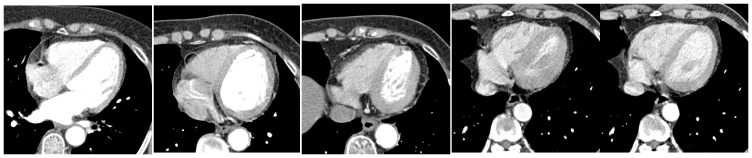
Some examples of cardiac MRI images used in our experiments.
